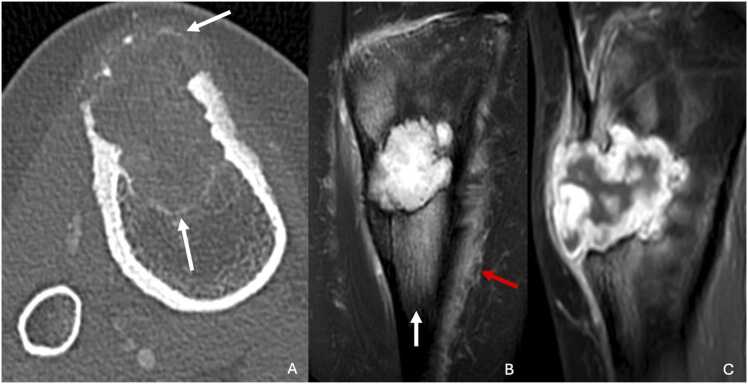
Chondromyxoid fibroma in a 19-year-old woman with painless swelling of the proximal third of the right leg for two years. A. CT image in the axial plan showing a cortical disruption of this eccentric osteomedullar lesion, with thin sclerosis margins (white arrows). B. Proton density-weighted fat-suppressed MR image in the coronal plane showing intense hypersignal with adjacent osteomedullar edema(white arrow) and soft tissue edema (red arrow). C. T1-weighted fat-suppressed contrast-enhanced MR image in the sagittal plane showing mainly peripheral enhancement without enhancement of central myxoid areas.
